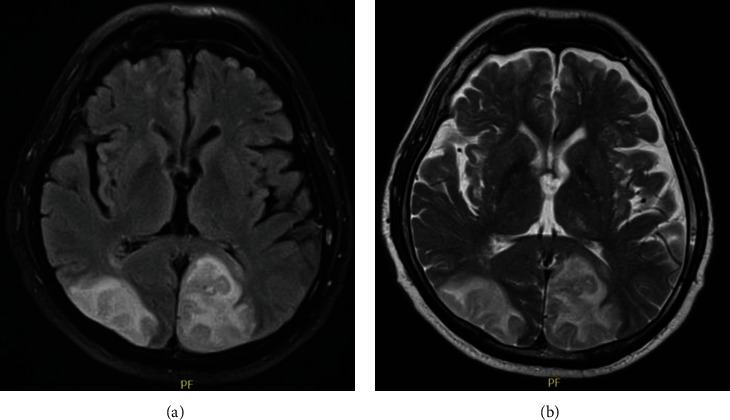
Brain MR of case 2. (a) Fluid-attenuated inversion recovery sequence showing extensive bilateral asymmetrical occipital hyperintensities in the occipital lobe and (b) its representation on T2-weighted sequence.
